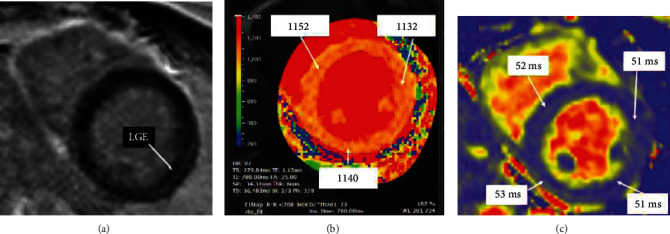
Tissue characterization on presenting cardiac MRI. Subtle stripe of epicardial LGE noted in the lateral wall (a). The native T1 relaxation time is diffusely elevated throughout the myocardium (ULN for this 1.5 Tesla magnet is 1010 ms), suggestive of diffuse inflammation (b). The native T2 relaxation time is mildly elevated throughout the myocardium and highest in the inferior wall (ULN: 50 ms), suggestive of edema (c). Abbreviations: LGE: late gadolinium enhancement; ULN: upper limit of normal.
